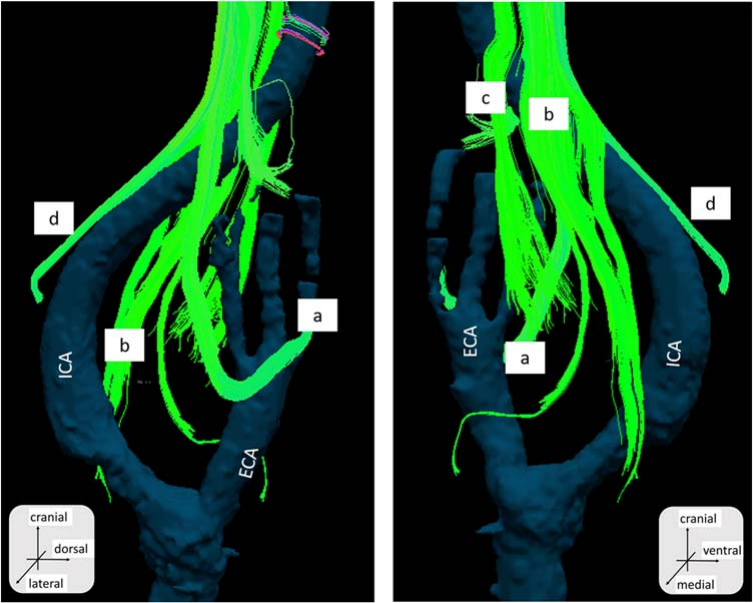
The diffusion tensor tractography fusion with artery structure obtained from CT. Right side neck. Sample number 1. a, The hypoglossal nerve; b, the vagus nerve; c, the glossopharyngeal nerve; and d, the spinal accessory nerve. ECA, external carotid artery; ICA, internal carotid artery [Color figure can be viewed at http://wileyonlinelibrary.com]
